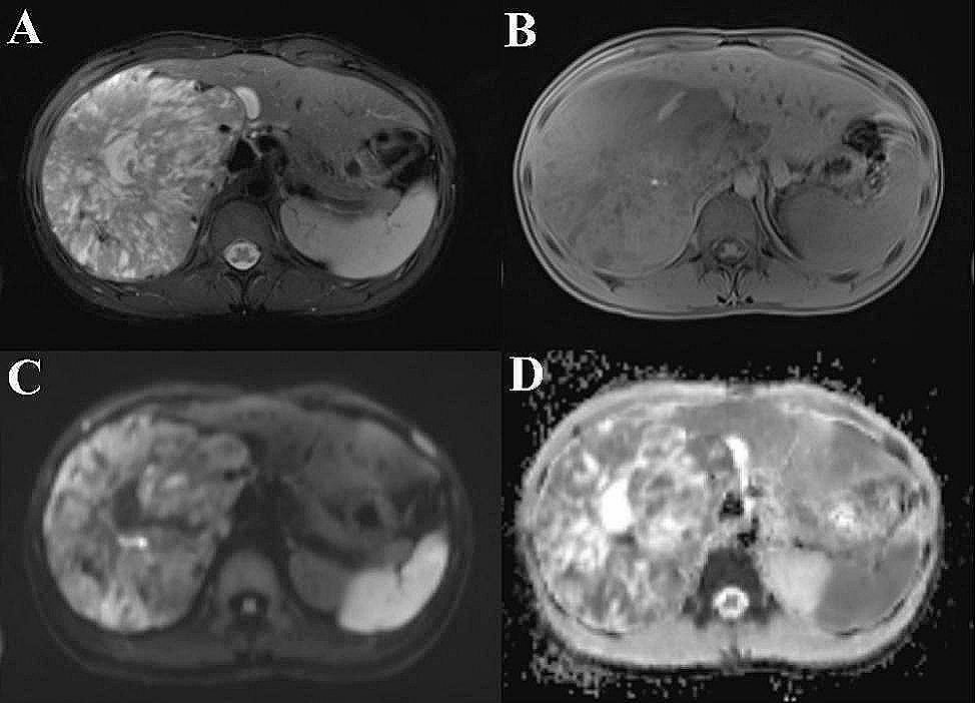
The provided figures depict an MRI scan showcasing a moderately malignant PEComa located in the right retroperitoneum. The image panels A, B, C, and D represent T1WI, T2WI, DWI, and ADC images, respectively
